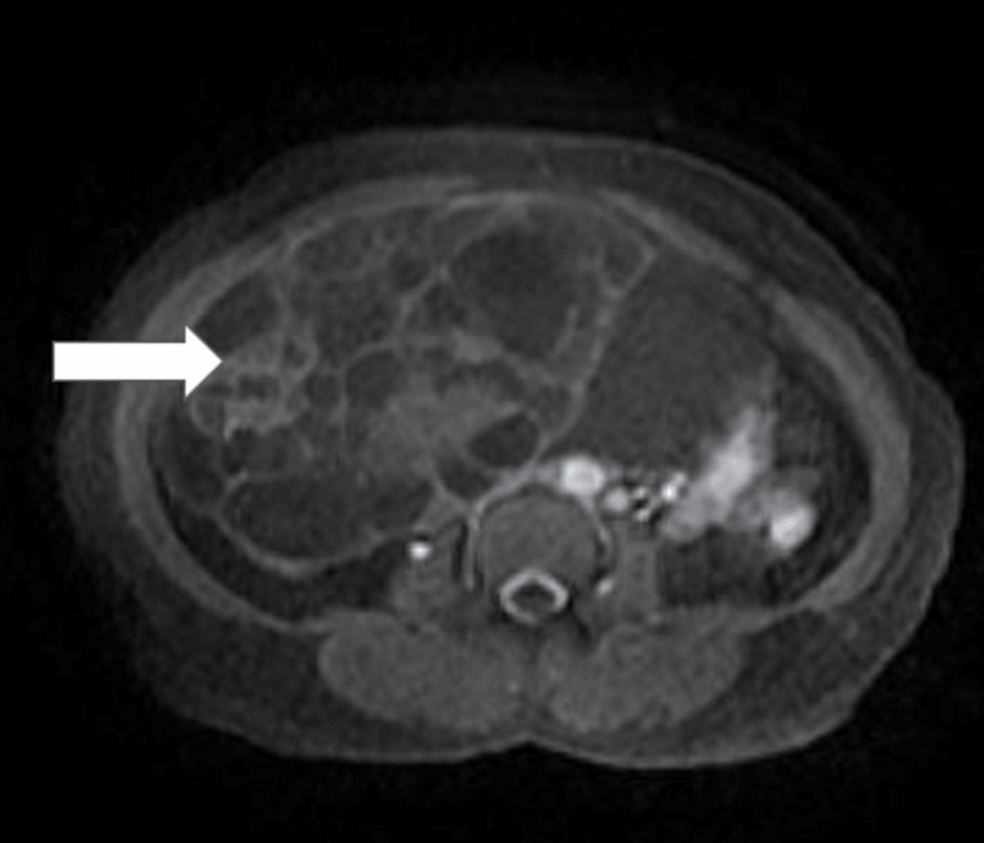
MRI axial post-contrast image showing an adnexal lesion with septal nodular enhancement (arrow).
